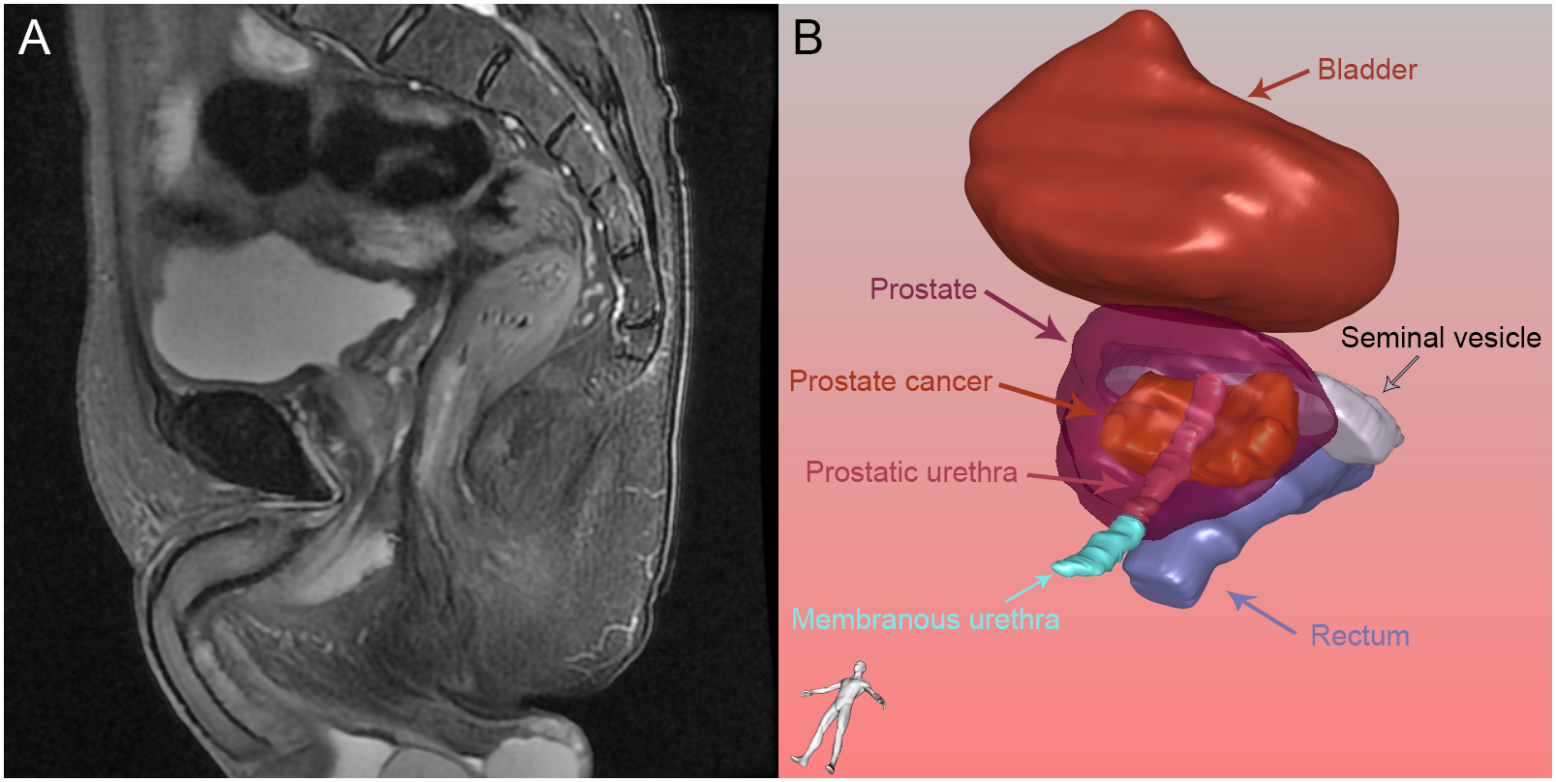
Prostate and its surrounding tissue: (A) Prostate and its surrounding tissue in magnetic resonance imaging; (B) Prostate and its surrounding tissue reconstructed by three-dimensional visualization.
